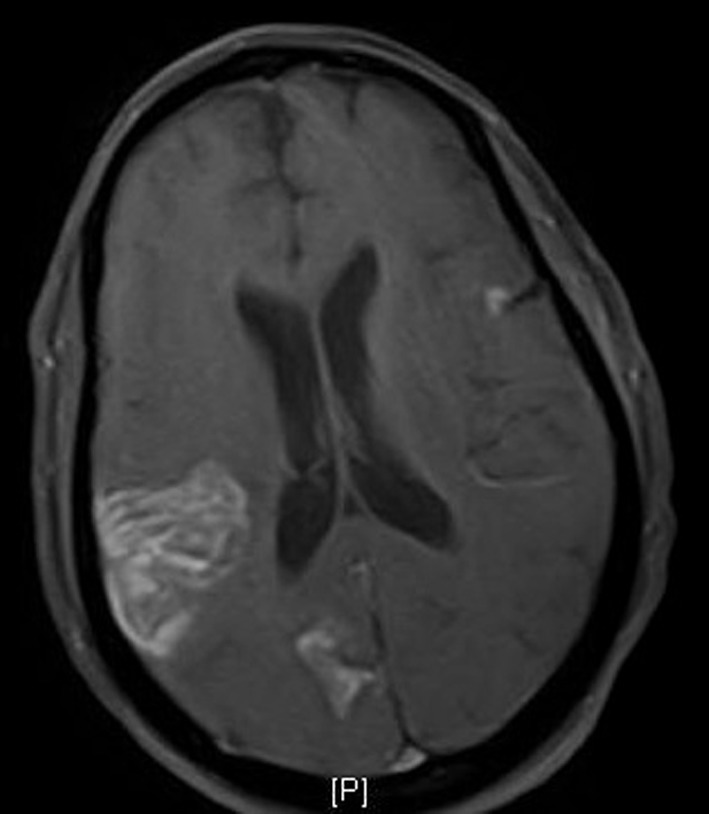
MRI of the brain shows acute and subacute infarcts in the frontal, parietal and occipital lobes.
